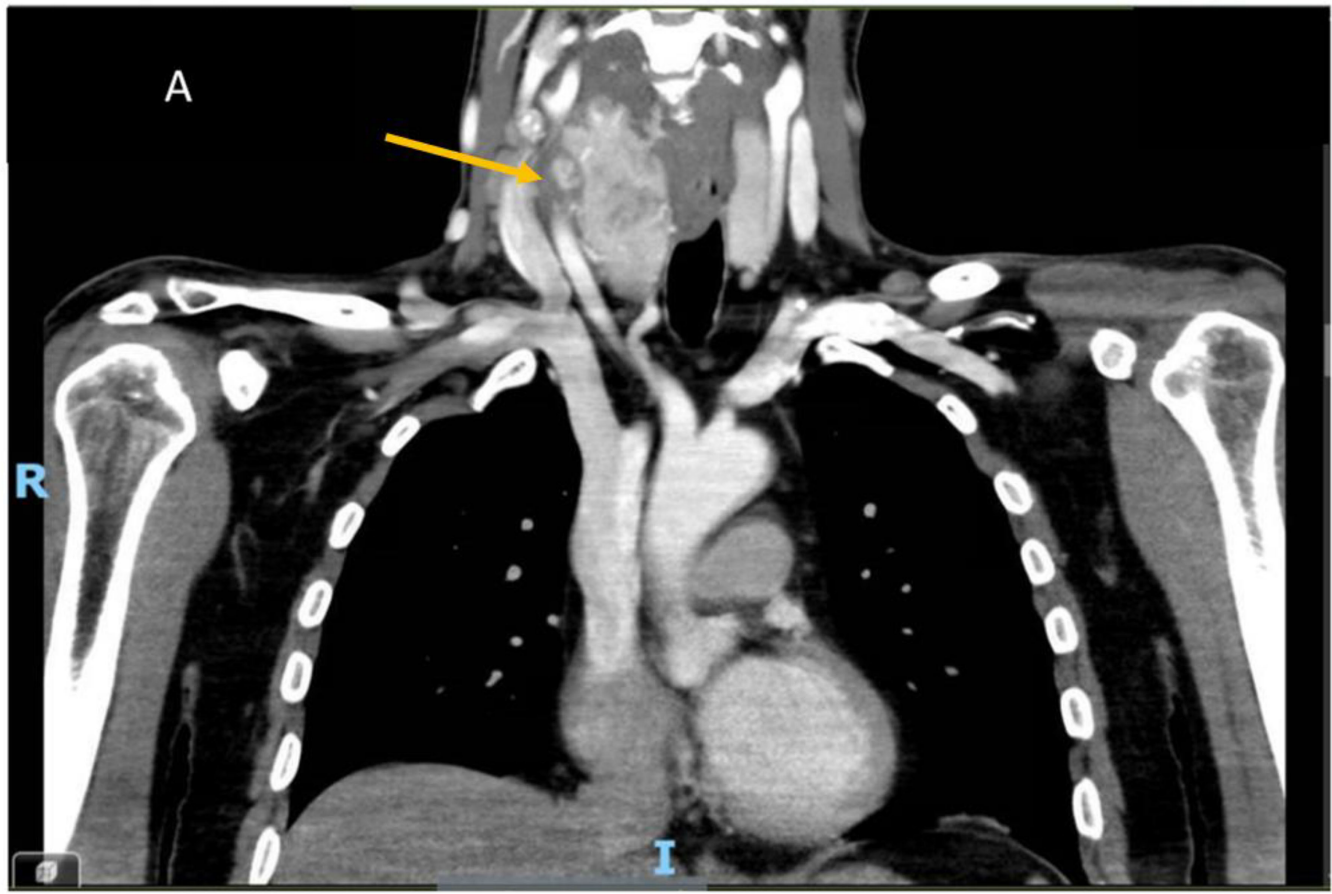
Contrast-enhanced computed tomography (CT) images of the neck and chest reveal a large nodule with areas of cystic degeneration within the right lobe of the thyroid gland. The yellow arrow indicates a tumor thrombus obstructing the right internal jugular vein (IJV).
